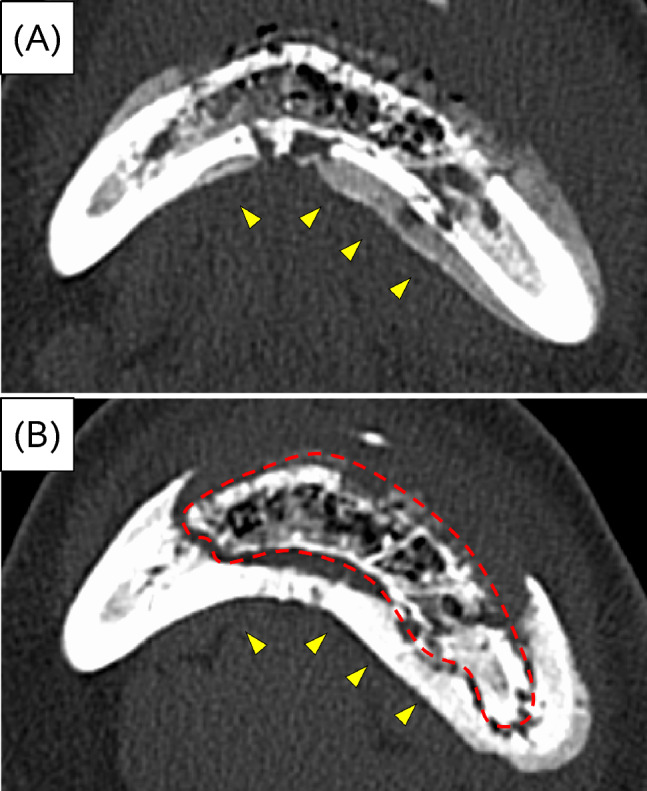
Temporal CT images of a patient with mandibular MRONJ. A First axial CT image. A discontinuous periosteal reaction is observed on the lingual side (yellow arrowheads). B Second axial CT image. 12 months after image (A), the lingual side of the periosteum is developed, and new bone formation is observed along the area of the periosteal reaction. The necrotic lesion appears as the region separated by MRONJ demarcation line (red dotted line)
